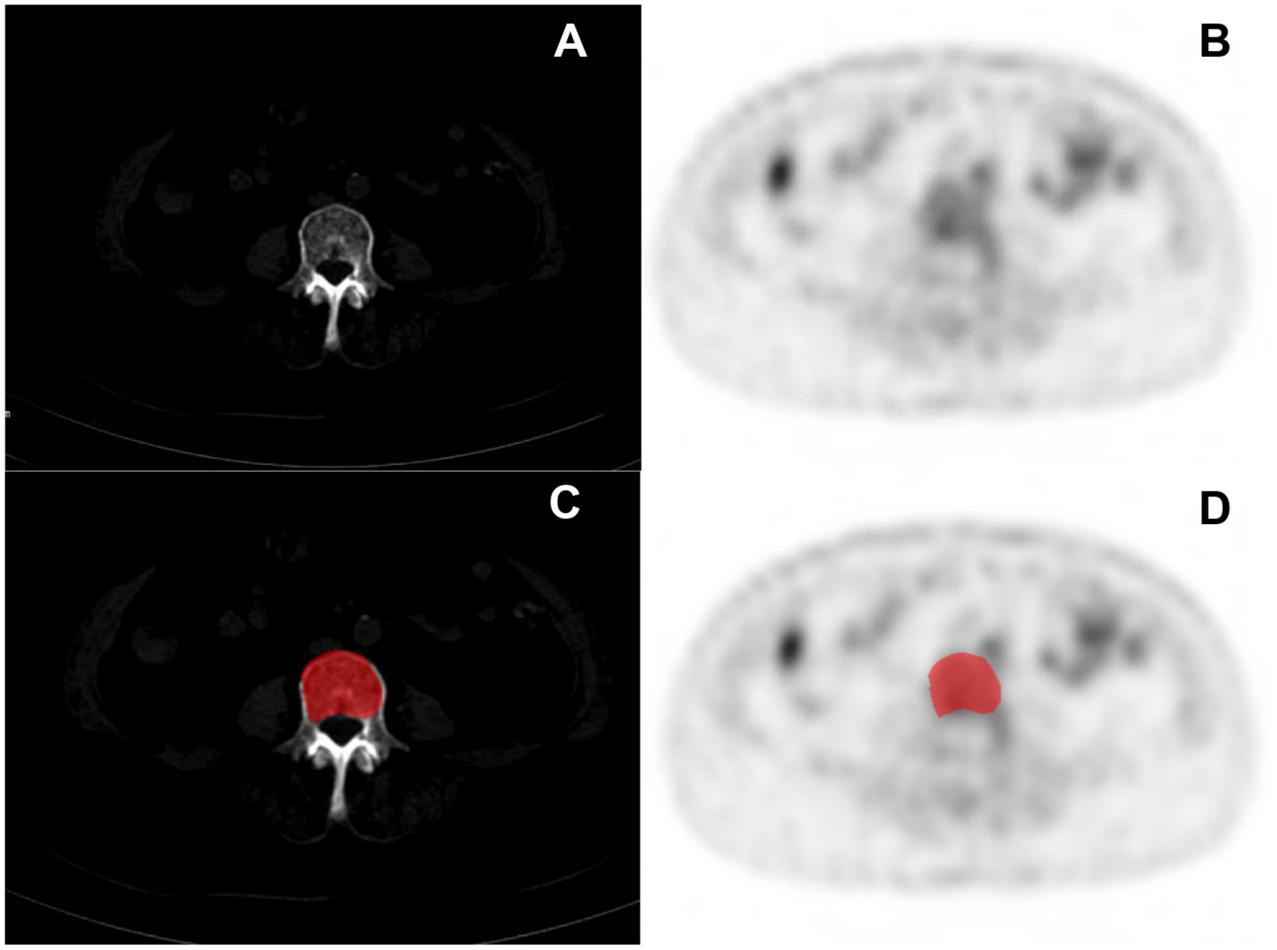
Clinical cases PET/CT images of multiple myeloma (MM) (A,B) and the delineation of the region of interest (ROI) (C,D).
